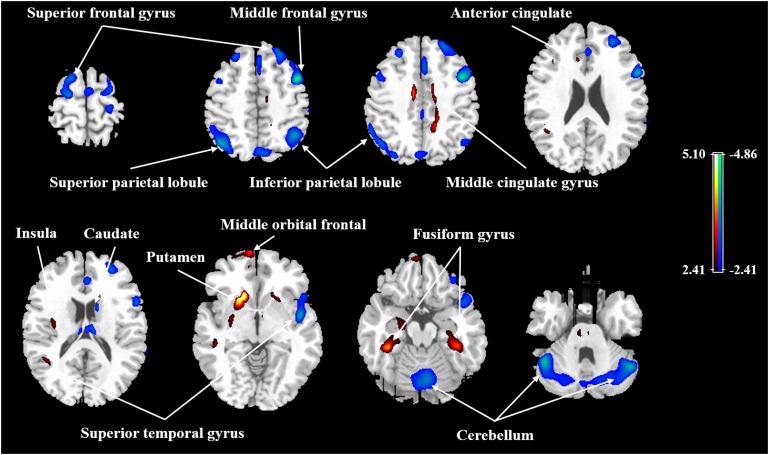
Age-related changes in relative CBF in all participants from regression analysis. Cold color indicates regions in which CBF negatively correlated with age, and warm color indicates regions in which CBF positively correlated with age. A threshold of 2.41 (*P* < 0.01) was used to overlay SPM maps onto a standard MRI brain template.
